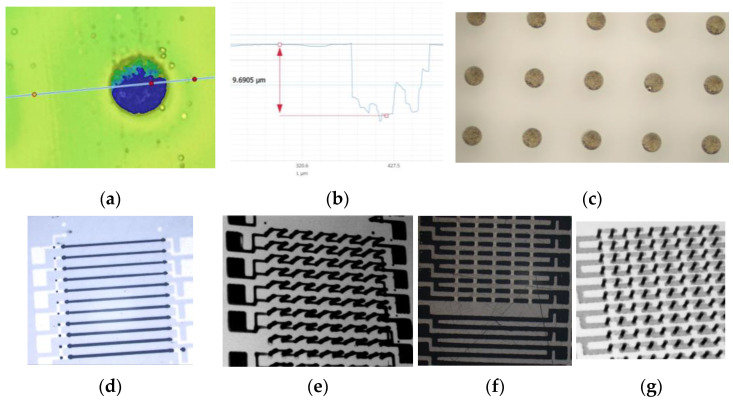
(a) The 3D images of paste-filled vias; (b) dishing of the paste-filled vias before thinning; (c) the top view of paste-filled vias after thinning; (d) the OM image of daisy chains using 3D printing to fill vias and surface wiring; (e) the X-ray image of daisy chains using 3D printing to fill vias and surface wiring; (f) the OM image of daisy chains using vacuum plugging to fill vias and surface wiring (thinning causes cracks in the glass); (g) the X-ray image of daisy chains using vacuum plugging to fill vias and surface wiring.
